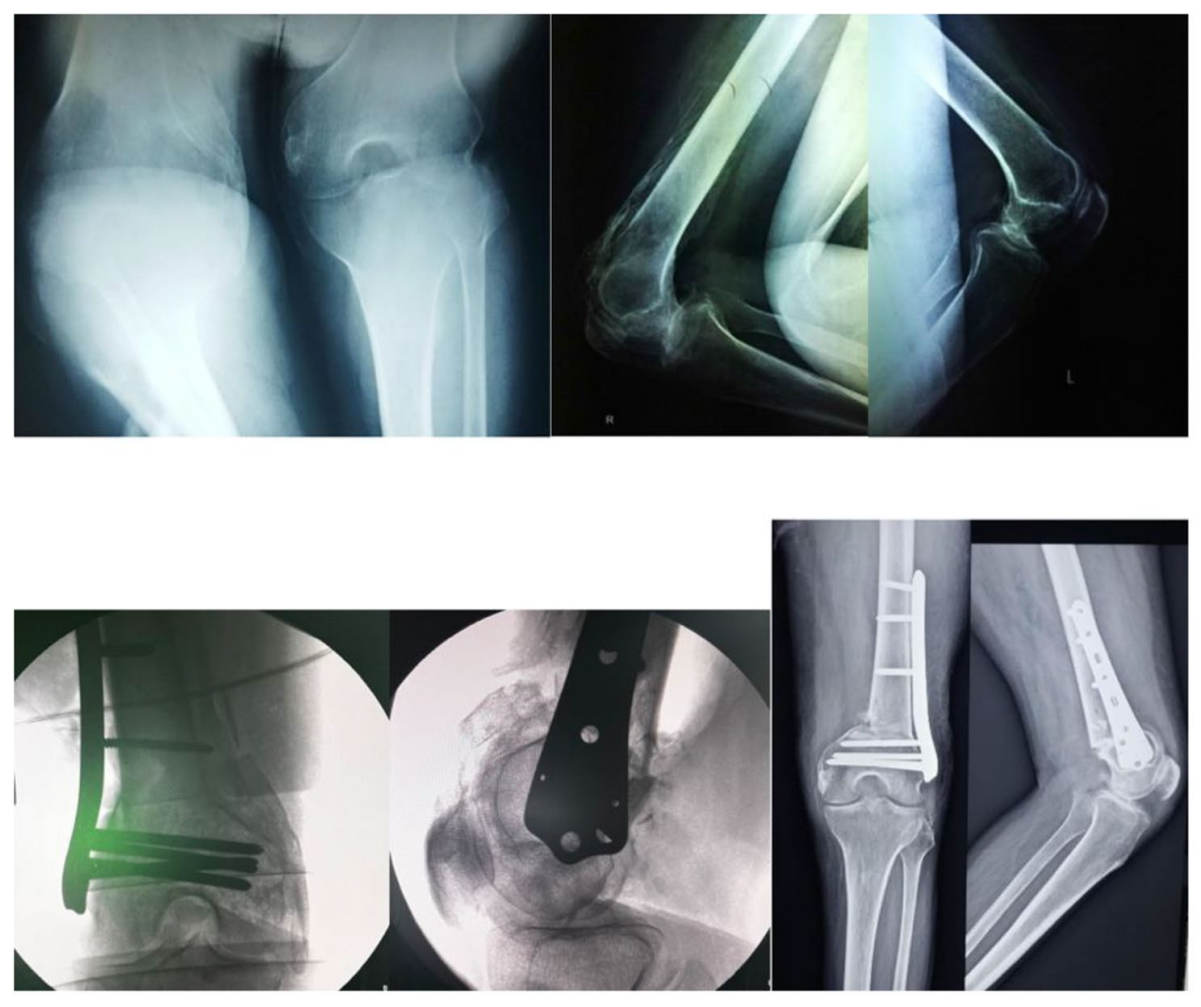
(Row: R → L) a. HO around Right & Left Knee (Antero-posterior & Lateral radiographs of Right & Left Knee) b. Post-operative radiographs after HO Excision & Deformity correction (Antero-posterior & Lateral radiographs of Right & Left Knee)
